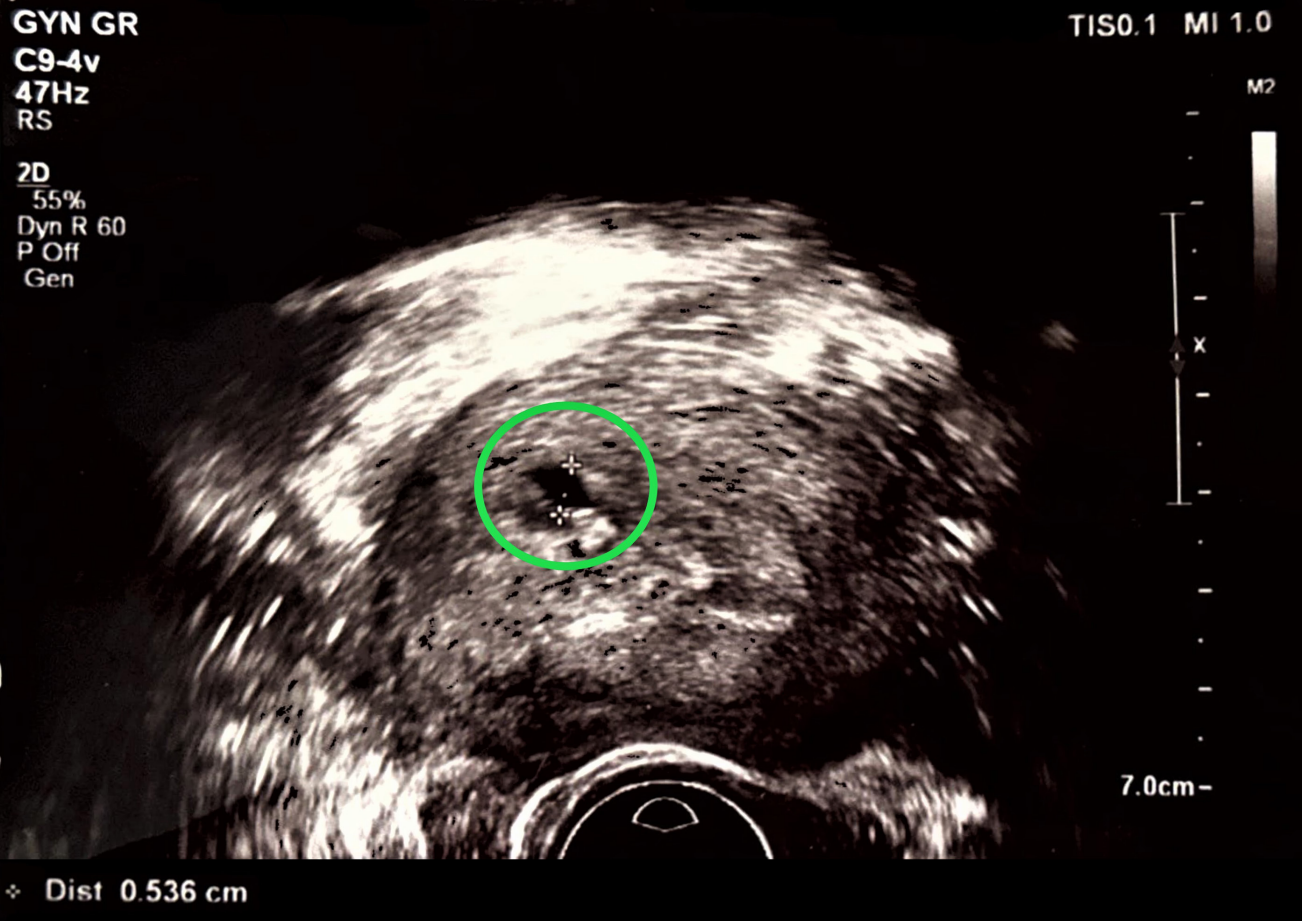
Transvaginal ultrasound findings from the scan performed at the 7th week of gestation. A small pseudo‐gestational sac without yolk sac is recognized at the fundus of the uterine cavity.
